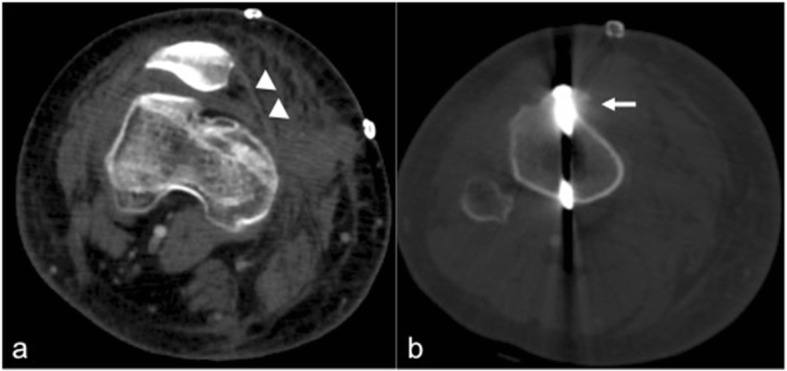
Knee CT images in the early post-operative period in a 19-year-old male with history of patellar maltracking. a Axial CT image demonstrating MPFL reconstruction (arrow heads). b Axial CT image showing tibial tuberosity transfer surgery with screw placement (arrow)
